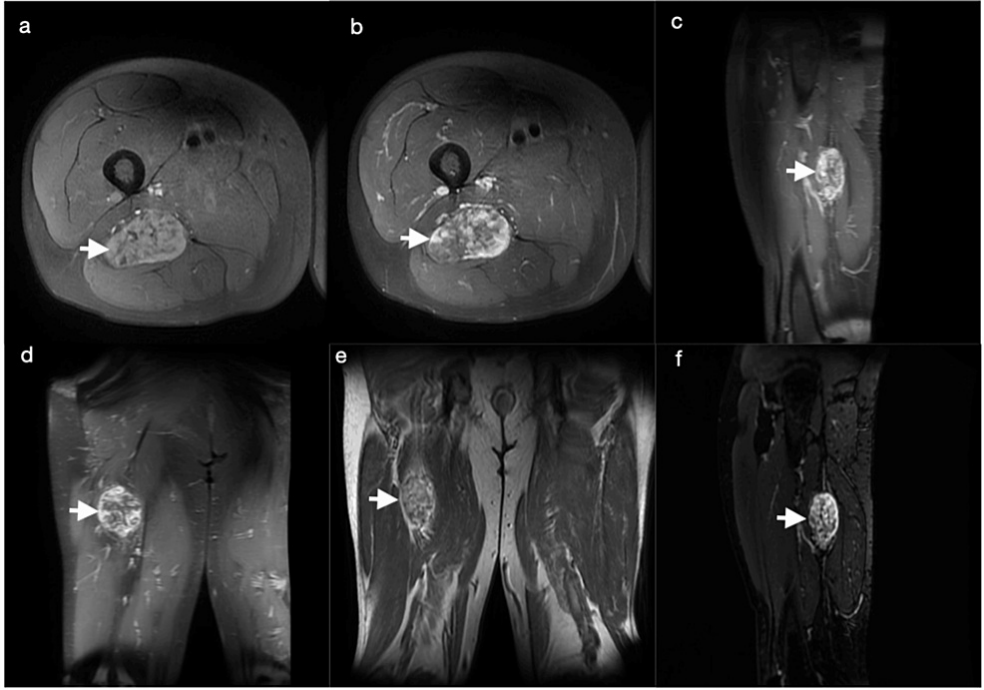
Magnetic resonance imaging of the lower extremity with and without contrast showed a well-circumscribed lesion with peripheral heterogeneous and central enhancement (a) T1; fat-suppressed; pre-contrast; TR, 793; TE, 10.36. (b) T1; axial; fat-suppressed with contrast; TR, 754; TE, 9.66. (c) T1; sagittal; fat-suppressed with contrast; TR, 810; TE, 9.40. (d) T1; coronal; fat-suppressed with contrast; TR, 697; TE, 10.09. (e) T2; bilateral coronal; TR, 4583; TE, 64.75. (f) T2; sagittal; fast spin echo; short tau inversion recovery; TR, 11532; TE, 64.51 TR, repetition time; TE, echo time
